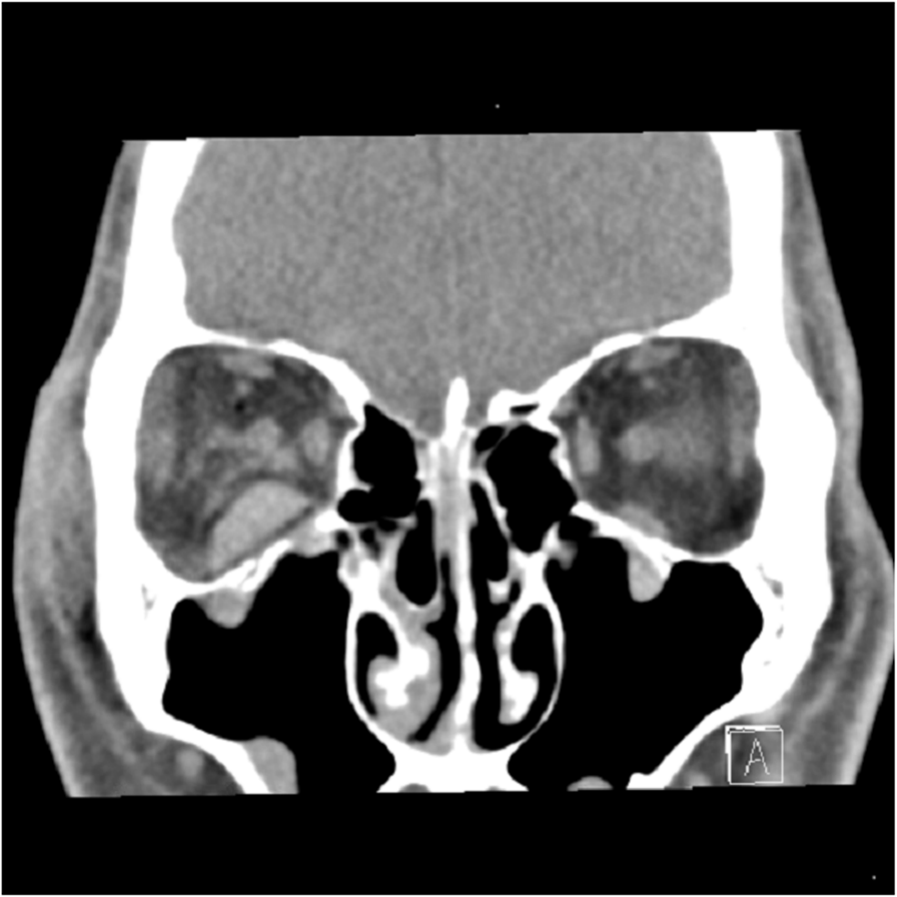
Computed tomographic image demonstrating retrobulbar hemorrhage at the inferior orbital rim after orbital blow-out fracture repair
